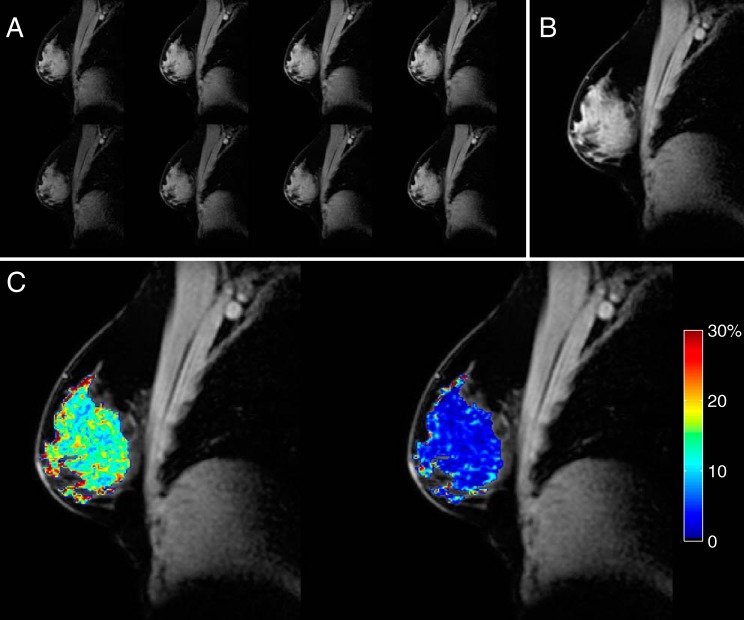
Quantitative magnetization transfer magnetic resonance imaging (qMT-MRI) data are shown for a typical subject (age = 26 years): the 8 magnetization transfer (MT)-weighted images, with pulse angles of 500° (top row) and 800° (bottom row) and offsets of 1, 2, 4, and 8 kHz (left to right) (A); the normalization image (offset frequency = 100 kHz and pulse angle = 800°) (B); and the corresponding pool size ratio (PSR) map (left) and map of the standard deviation (SD) of the PSR values (right) (C) calculated during the fitting process. The mPSR ± SD for this subject and scanning session was 14.5% ± 5.9%.
